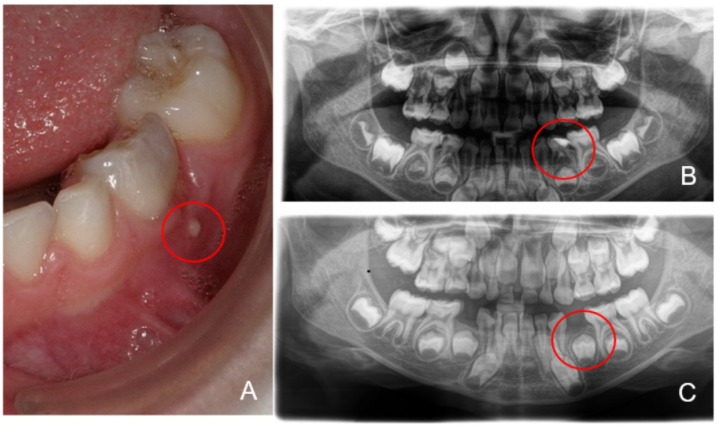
Patient CHD06 with severe early childhood caries (S-ECC) and fistula (marked by the red circle) of decayed tooth 74 (A), radiographic showing parodontitis periapicalis chronica as a result of endodontic infection with the need for extraction of tooth 74 (marked by the red circle; B) and the post-therapy recall after extraction tooth 74 after three years (marked by the red circle; C).
